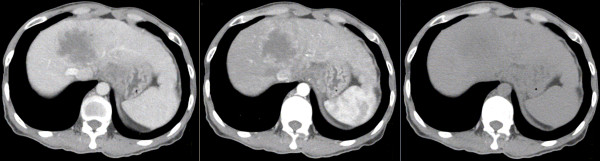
Three phases of CTs for the same patient. Left: portal venous phase (PVP) CT; Middle: hepatic arterial phase (HAP) CT; Right: non-enhanced CT.
